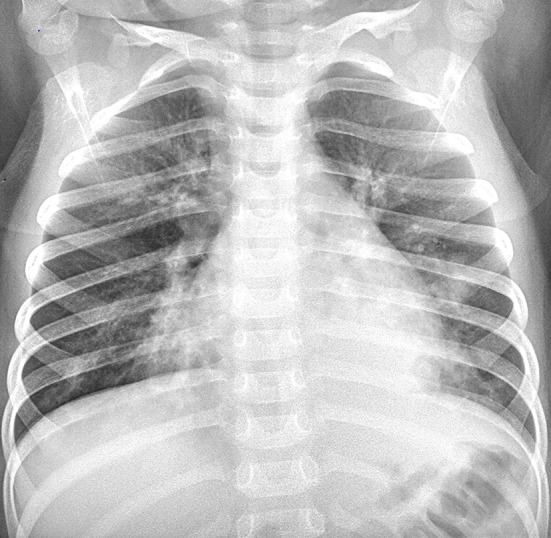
Dynamic changes on chest X-ray (CXR) and CT scans of our 2 patients. Patient 2: CXR showed bilateral patch ground glass opacities in both lungs on hospital day (HD) 1.
